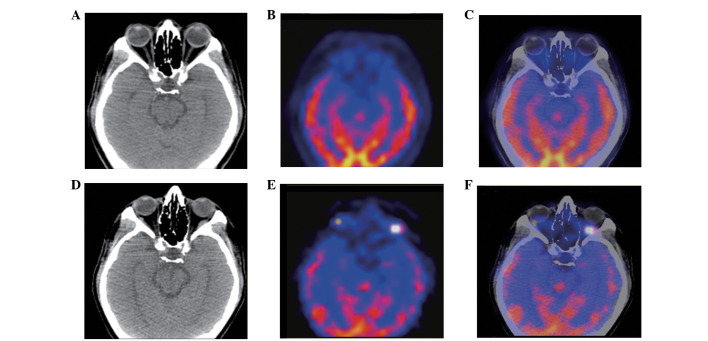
Lateral rectus lesion in the left extra-ocular muscles demonstrated by (A and D) computed tomography (CT), (B and E) Fluorodeoxyglucose-positron emission tomography (FDG-PET) and (C and F) the fusion of CT and FDG-PET. (A–C) Normal appearance of the pretreatment examination. (D–F) Twenty-four weeks after the initiation of treatment with sorafenib, a new left lateral rectus lesion was evident in the follow-up examination. This resulted in an objective response of progressive disease (PD) according to response evaluation criteria in solid tumors (RECIST) 1.1. (E) The FDG-PET image reveals a lesion with significant metabolic activity, with mean and maximum standardized uptake values of 4.8 and 10.0, respectively. (D) The CT image and (F) the fusion image verified the FDG-PET findings, showing the same leison with a dimension of 1.2×0.6 cm and a CT value of 42 Hounsfield units (HU).
